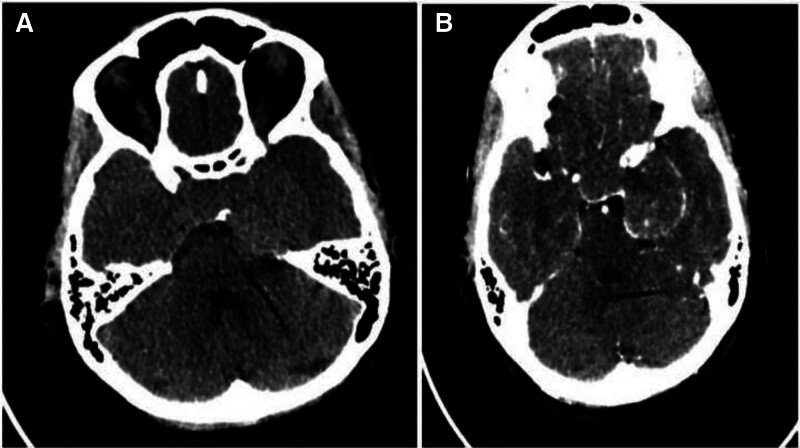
CT scan of the mass including non-contrasted (A) and contrasted images (B), these showed isodense mass centered in the left cavernous sinus with no bony destruction. Arterially enhancing components are noted within the mass. CT = computed tomography.
